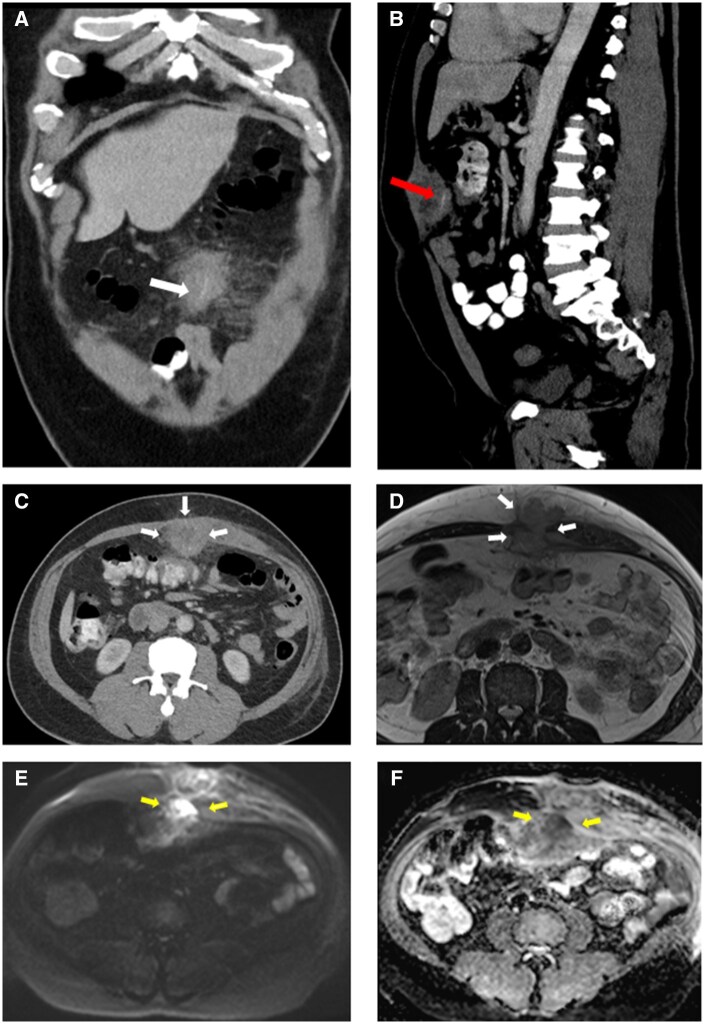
Case 1. Coronal (A), sagittal (B), and axial (C) CT with PV contrast as well as axial T1 MRI images (D) showing the abdominal wall phlegmon (white arrows) and a linear hyperdense fish bone (red arrow) penetrating the transverse colon and extending into the left rectus sheath. DWI B800 (E) and ADC map (F) show areas of marked diffusion restriction within the inflammatory mass.
